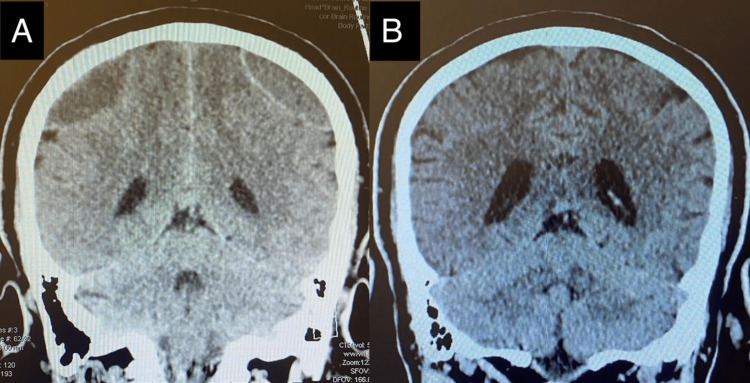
Coronal CT scan of the brain: (A) bilateral parietal hypodense fluid collection and (B) the resolution of bilateral EDH. CT: computed tomography, EDH: epidural hematoma
